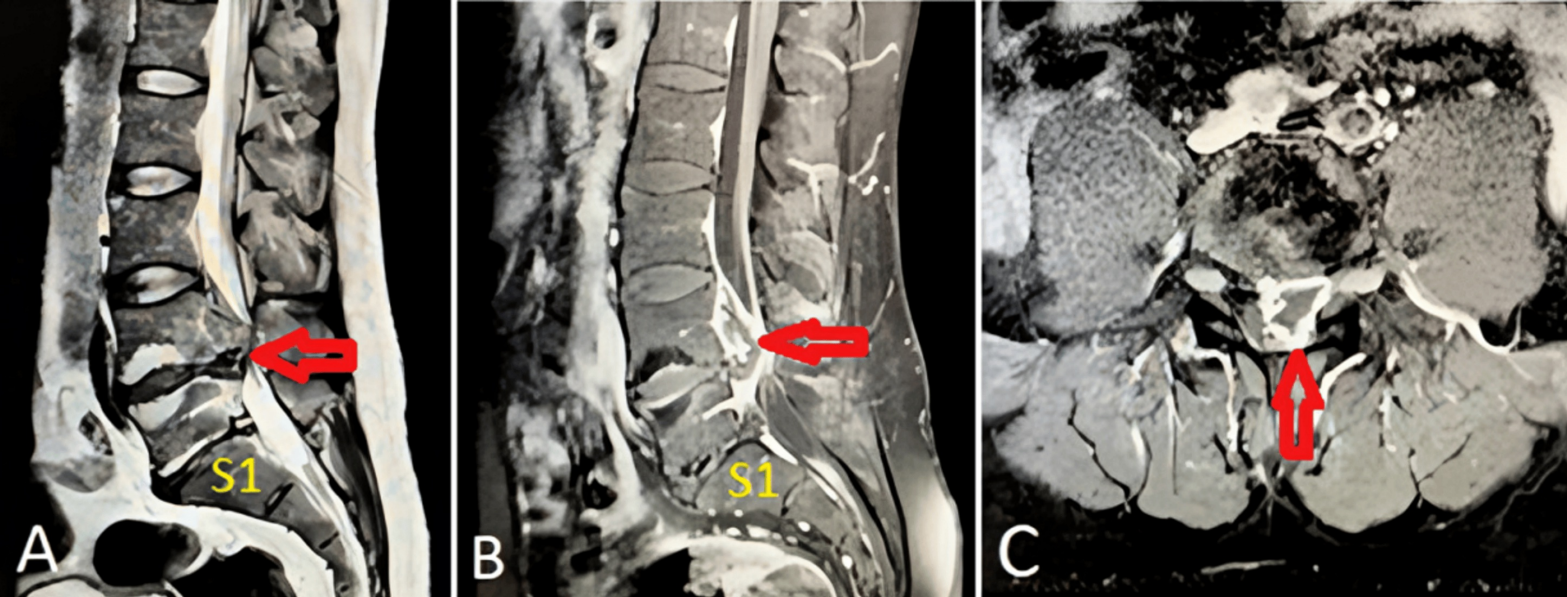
(A) Magnetic resonance imaging of lumbar spine in T2 sagittal without contrast identifies a symmetric epidural mass lesion (arrow) measuring 4x1.9x1.7 cm (CCxAPxT) at the epidural space of L4/L5. It shows T2W hyperintense signal and slight signal difference with the original disc. No bone erosion or destruction and the mass lesion compresses the L5 root in the left. The differential diagnosis includes epidural abscess and spinal tumor. (B and C) Magnetic resonance imaging of lumbar spine in T1 sagittal and axial with gadolinium contrast identifies that the L4/L5 epidural mass lesion shows with peripheral enhancement (arrow). CCxAPxT: Craniocaudal × Anteroposterior × Transverse
